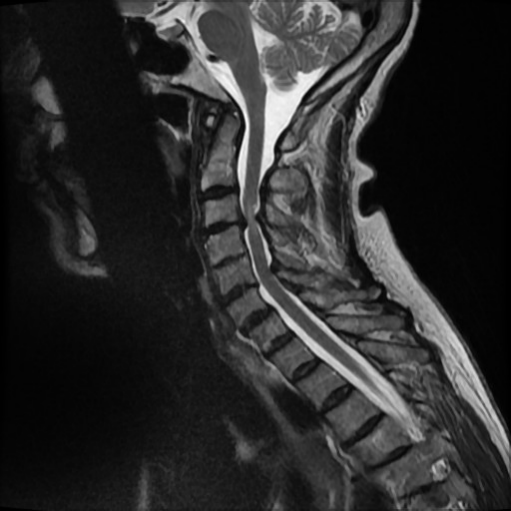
Sagittal MRI of cervical spine.
